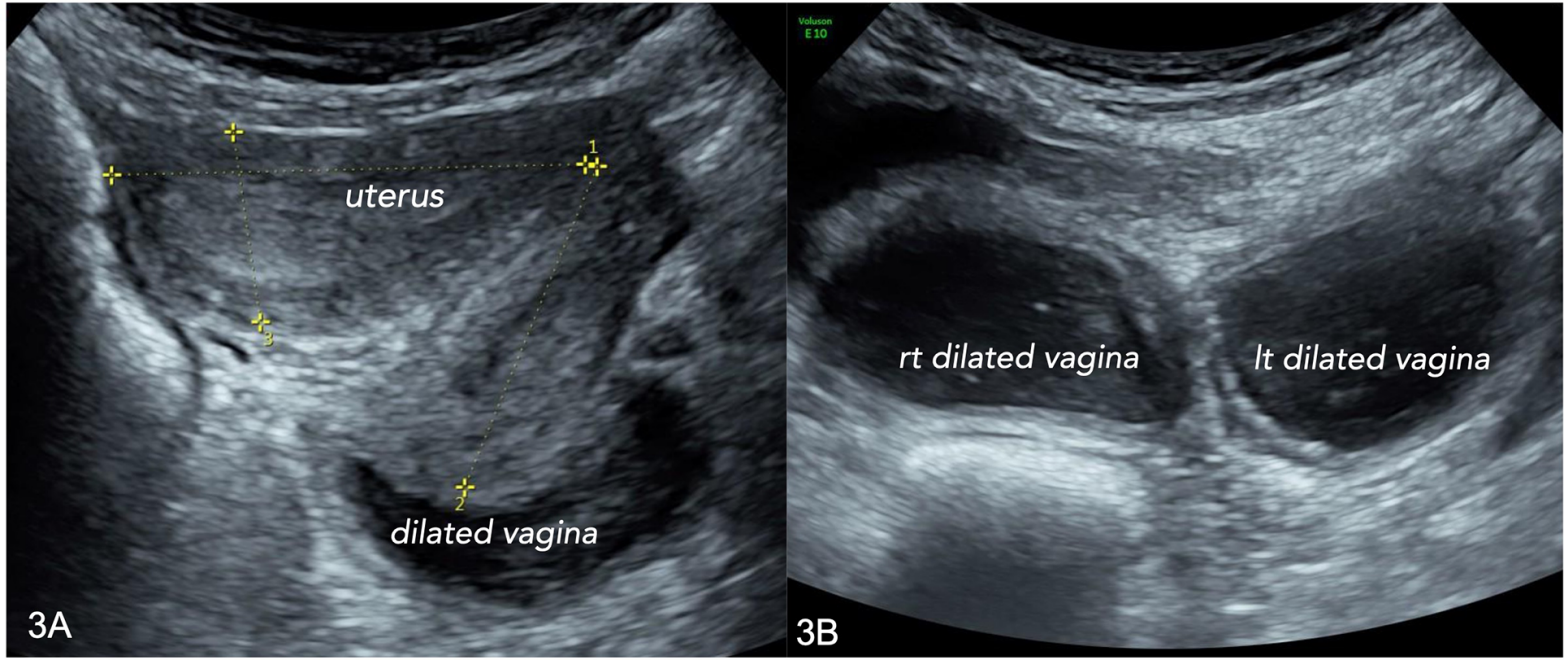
Transabdominal ultrasound. (A) right uterus and vagina, longitudinal scan; (B) both vaginas, dilated aspect, with “low-level” content, in in a transverse scan.
